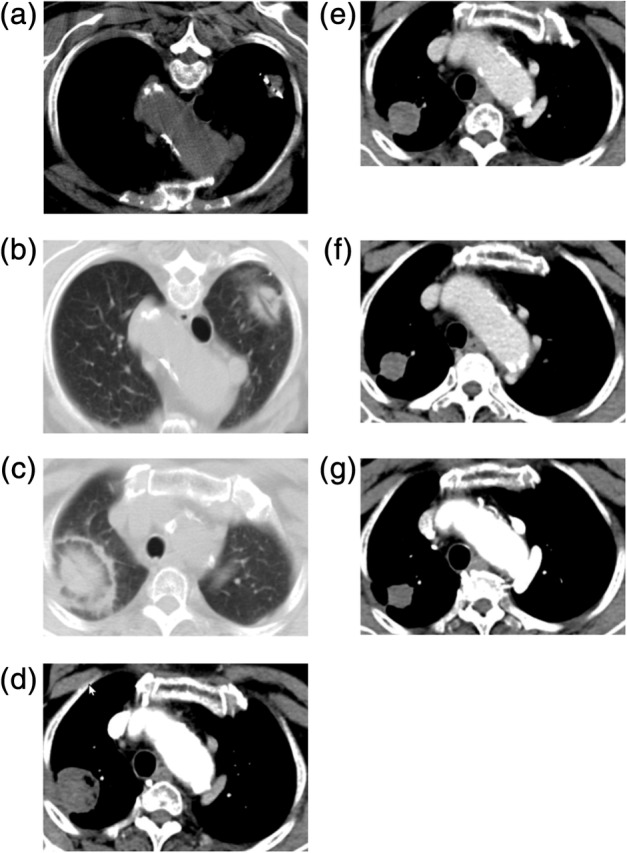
CT of an 80‐year‐old woman with right upper lobe adenocarcinoma at stage IA (cT1aN0M0). (a) The MWA antenna is shown centrally positioned within the tumor. (b) Ground‐glass opacity around the tumor and cavity in the core was observed in the image obtained immediately postablation. (c) The axial CT with lung window showed expected thermal damage around the target lesion, without pneumothorax, 24 hours postablation. (d) The enhanced CT image at one month after MWA; the size of the ablation zone was larger than the target tumor but was not enhanced; (e–g) The images at six, 12, and 19 months after MWA; the ablation zone shrank continuously and remained nonenhanced. CT, computed tomography; MWA, microwave ablation.
